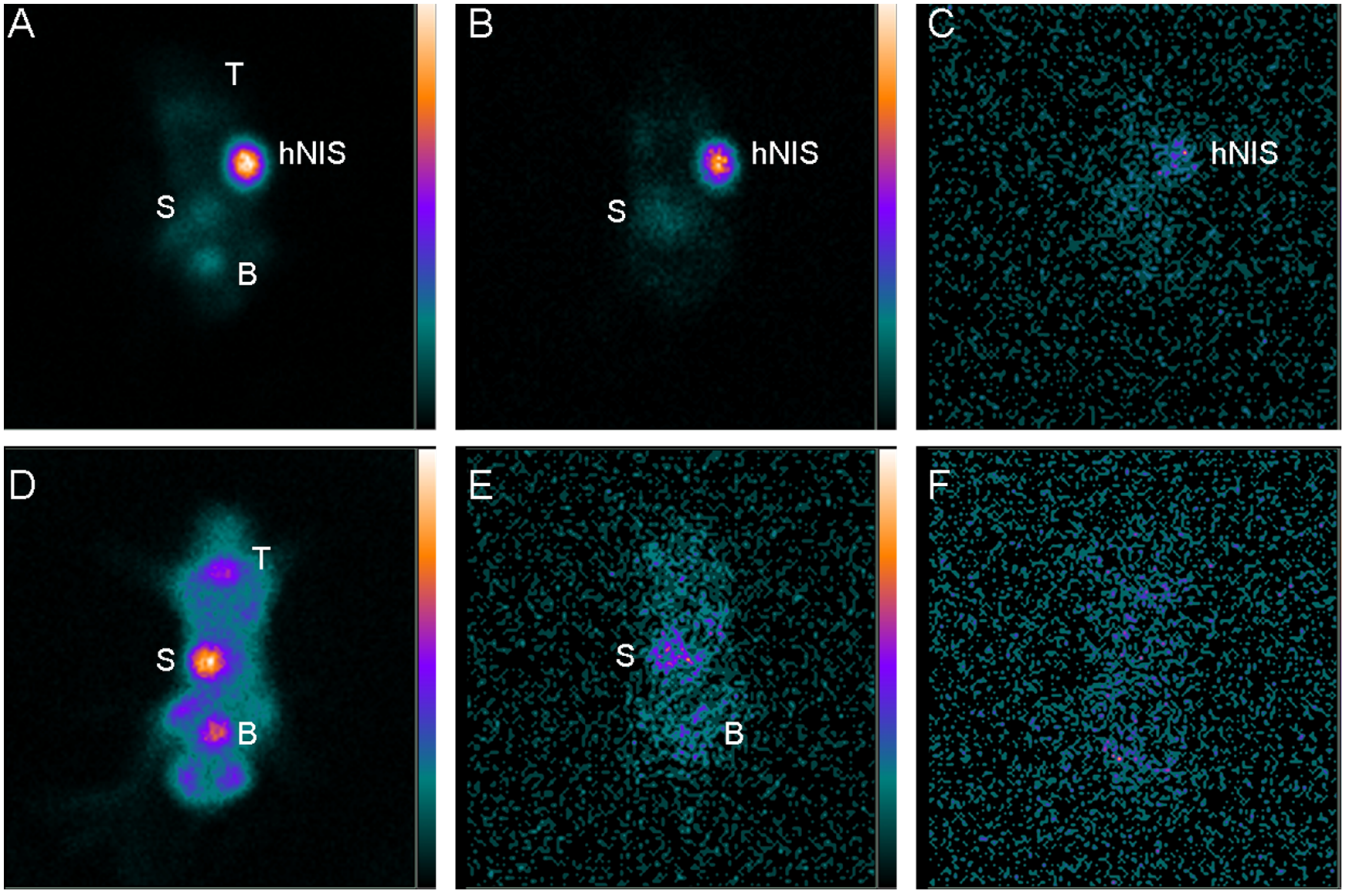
Whole-body scintigraphic images (anterior view) of hNIS-expressing U87-hNIS tumors (the first line, right axilla) or U87-0 tumors (the second line, right axilla) at 1-hour (A, D), 24 hours (B, E), and 48 hours (C, F) after intravenous injection of ^188^Re. T = thyroid; S = stomach; B = bladder; hNIS = hNIS-expressing tumor.
